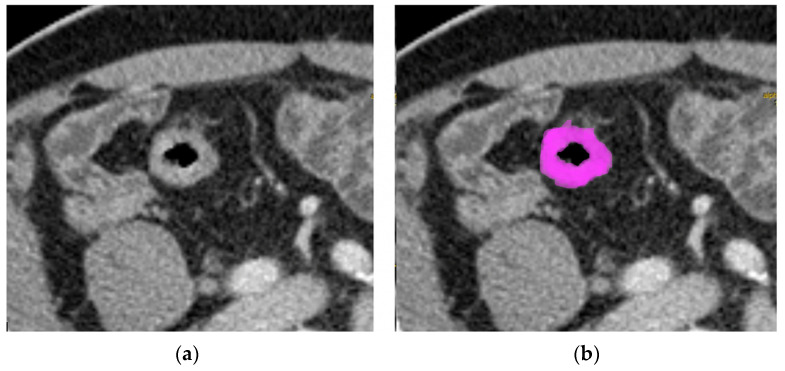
Tumor tissue in the axial CT portal venous phase images in locally advanced CRC in a 77-year-old man (a). The pink area represents the regions of interest; the procedure was applied for every single slice where the tumor was detectable, obtaining a 3D-ROI (b).
